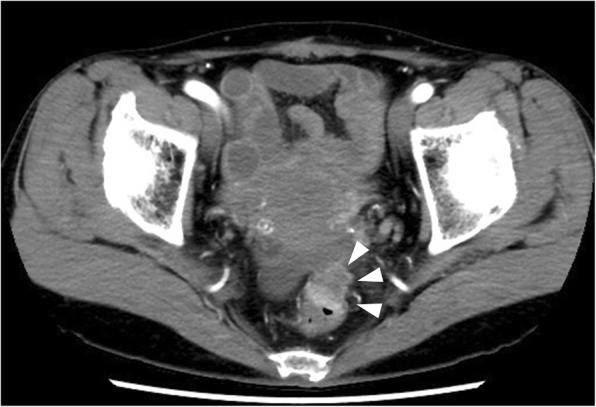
Computed tomography scan of the patient’s abdomen and pelvis showing a tumor 20 mm in size along the middle rectal wall and expanding into the pelvis (arrowhead)
